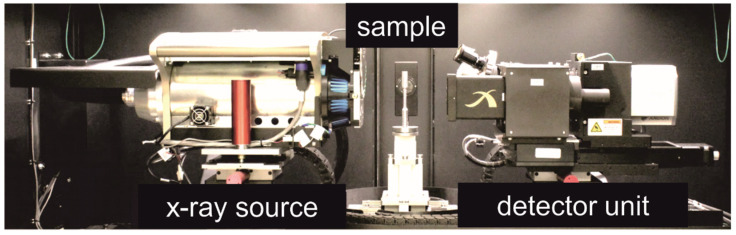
Measuring unit of the µ-CT Xradia 520 versa [22].
